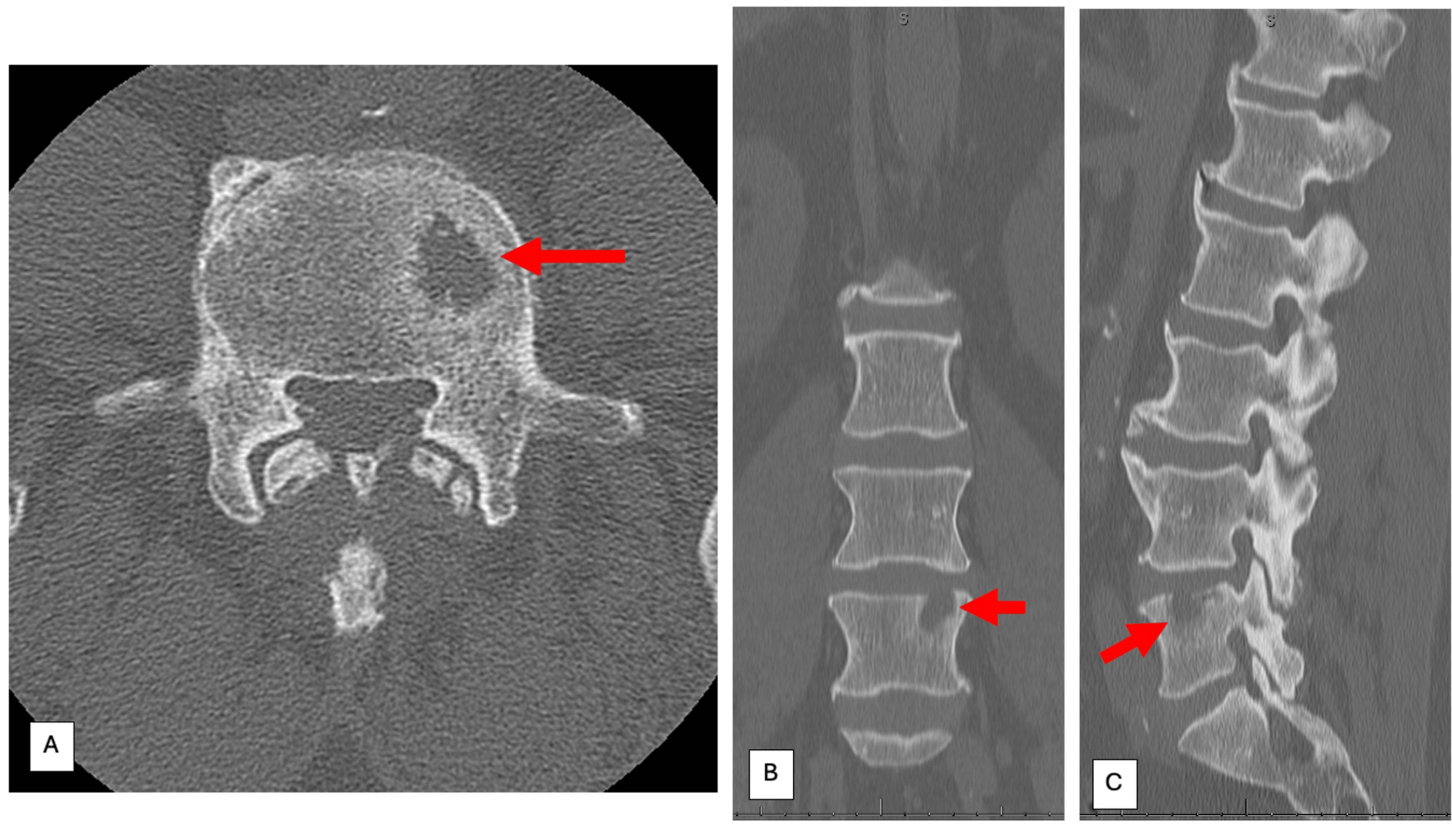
Non-contrast computed tomography of the lumbar spine obtained as part of the initial evaluation for neurogenic claudication, approximately 2.5 years after the patient's diagnosis of prostate cancer and approximately 1.5 years prior to his presentation to our office. This was the earliest image that demonstrated an L5 vertebral body lesion. At that time, the hypodense lesion measured 18.7×12.6×12 mm. Figures 2A, 2B, and 2C demonstrate the L5 vertebral body and associated lesion on axial, coronal, and sagittal cuts, respectively. Red arrows denote the L5 vertebral body lesion on axial, coronal, and sagittal computed tomography imaging.
